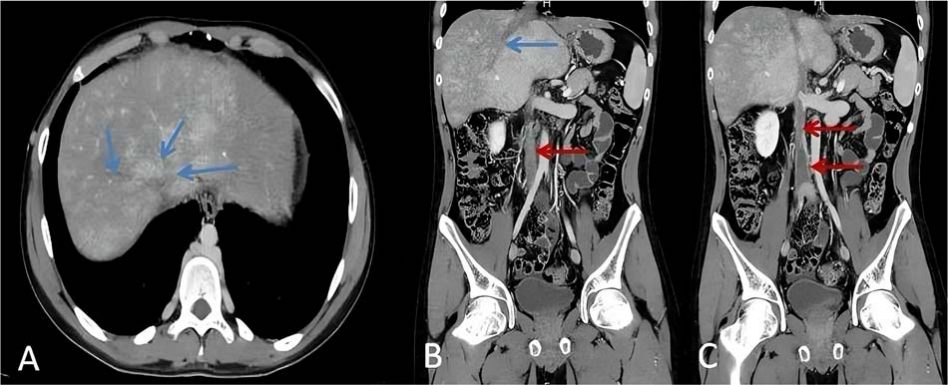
Axial and coronal CT image in a patient with BCS shows non-opacified hepatic veins (blue arrows, A and B). Coronal CT image in a patient with thrombosis of IVC seen as filling defect causing expansion of the lumen (red arrows, B and C).
